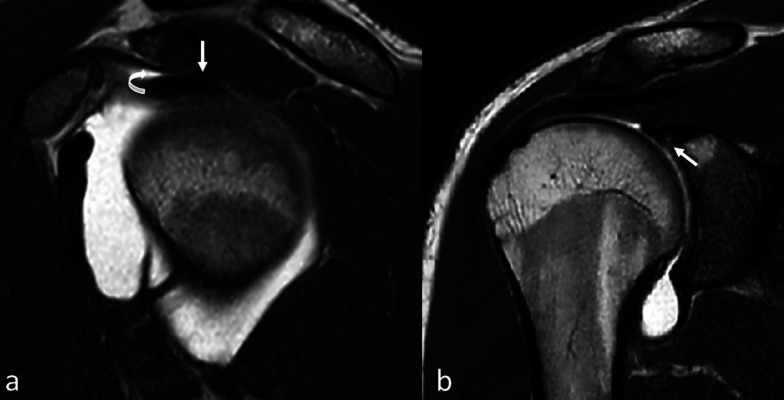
a MR arthrography, sagittal SE T1-weighted. In MESO-PA anatomical variant, long head biceps tendon is partially fused to the inferior surface of the supraspinatus tendon (arrow). Contrast agent outlines acute angle between the anterior portion of the long head biceps tendon and the inferior surface of the supraspinatus (curved arrow). b Same patients as in a. MR arthrography, coronal SE T1-weighted. MESO-PA anatomical variant is associated with SLAP lesion type I. MESO-PA anatomical variant is associated with SLAP lesion type I (arrow)
